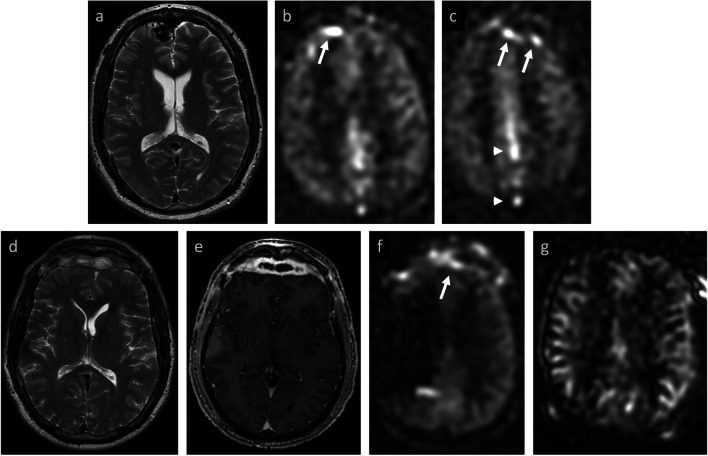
Example of ASL false positive in dAVF patient who developed intracranial infection after surgical resection. Baseline MRI: right frontal parafalcine dAVF status post prior embolization (a) with ASL signal along the frontal convexities (white arrows) and superior sagittal sinus (white arrowheads) suggestive of residual AV shunting (b, c). Post-resection MRI: post-operative course was complicated by subdural empyema seen on axial T2 (d) and post-contrast imaging (e). Elevated ASL signal along the frontal convexities and extracranial soft tissues (white arrow, f) was interpreted as residual AV shunting by both readers, but in retrospect was likely secondary to infection, given resolution on subsequent MRIs (g). Note that ASL signal dropout within the right lateral hemisphere is due to a VP shunt. Post-resection DSA: confirmed obliterated dAVF (not shown)
